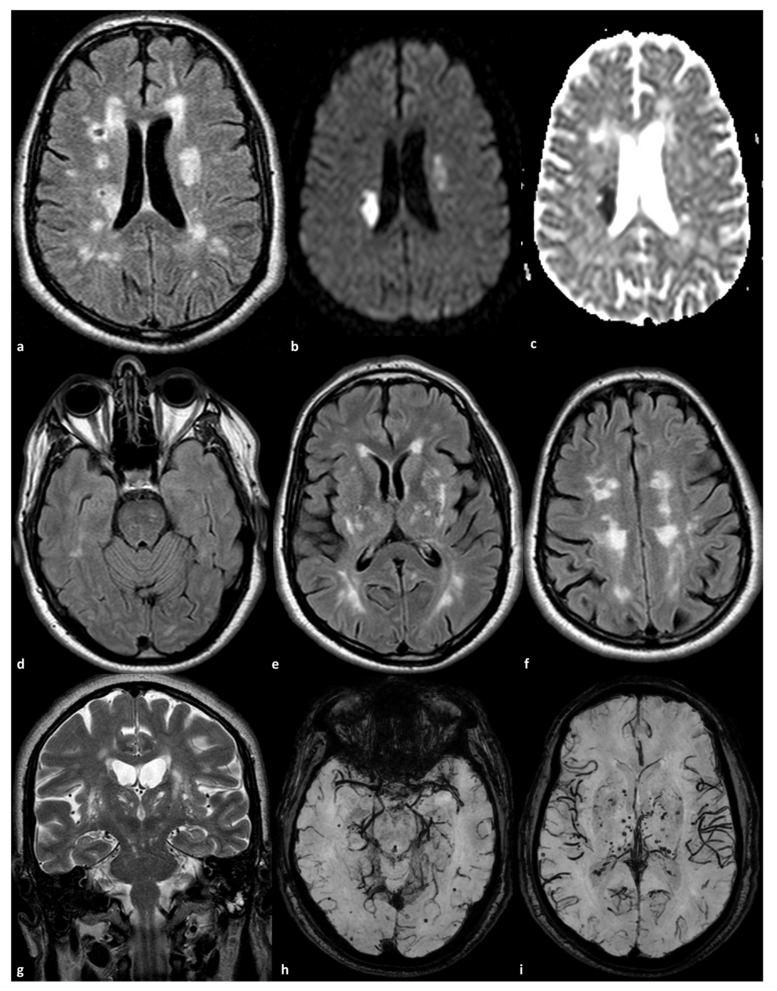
Brain MRI of a 56-year-old man with a homozygous *NOTCH3* mutation p.R1231C (Ex22) and a past history of arterial hypertension. The diagnosis was performed after admission to the Stroke Unit due to acute stroke (panel (a): axial FLAIR; panel (b): DWI; panel (c): ADC map) with a dual DWI-positive subcortical lesion in the corona radiata of right and left hemispheres. The right stroke was the most recent one. The remaining brain in FLAIR (panels (d–f)) showed symmetrical WMHs sparing the anterior temporal pole and involving the external capsule, the basal ganglia, the subcortical white matter in the occipital lobes, and the centrum semiovale. EPVSs in the basal ganglia are evident in T2WI in coronal view (panel (g)). Several subcortical lobar (panel (h)) and deep (panel (i)) cerebral microbleeds are present in susceptibility-weighted imaging.
